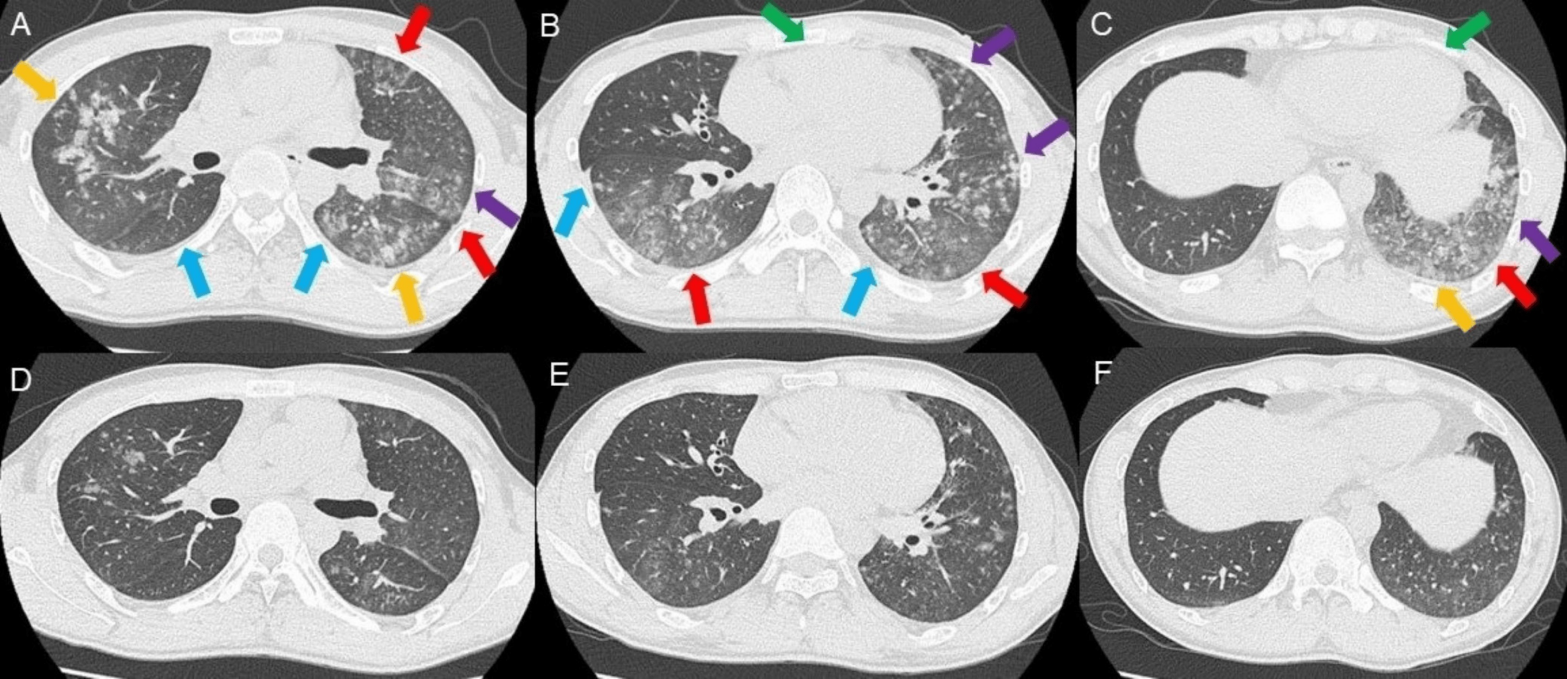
Time-course change in findings of computed tomography (CT). (A–C) CT on the day of admission. Ground-glass opacities (red arrows), granular shadows (purple arrows), and nodular infiltrative shadows (orange arrows) in both lung fields. Although there is a small amount of bilateral pleural effusions (light blue arrows), cardiac enlargement is not observed (green arrows); (D–F) CT on the 3^rd^ day of admission showing improvement in the pneumonic shadows in both lung fields and a decrease in bilateral pleural effusions.
